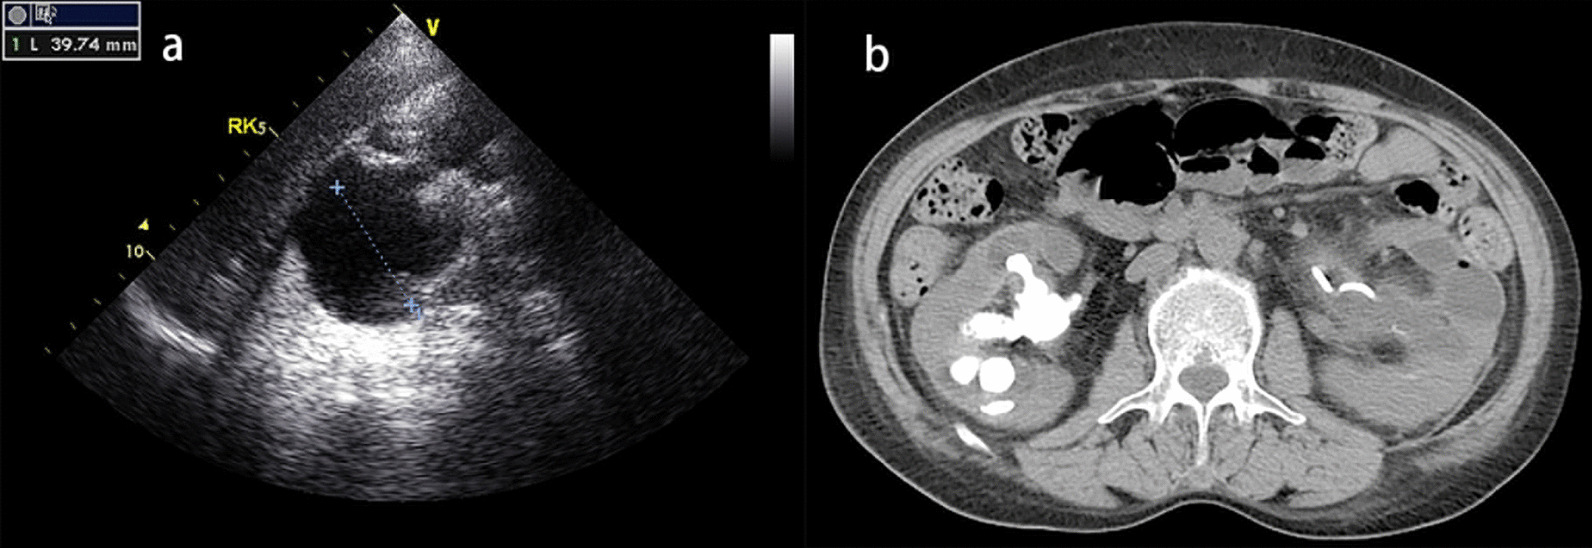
Mild complication after PCNL in 57-year-old woman who underwent CT scan and nephrosonography preoperatively. a Sonagraphic view demonstrated anteroposterior diameter (APD) of right kidney was 39.74 mm, severe hydronephrosis. b CT view of staghorn calculi
